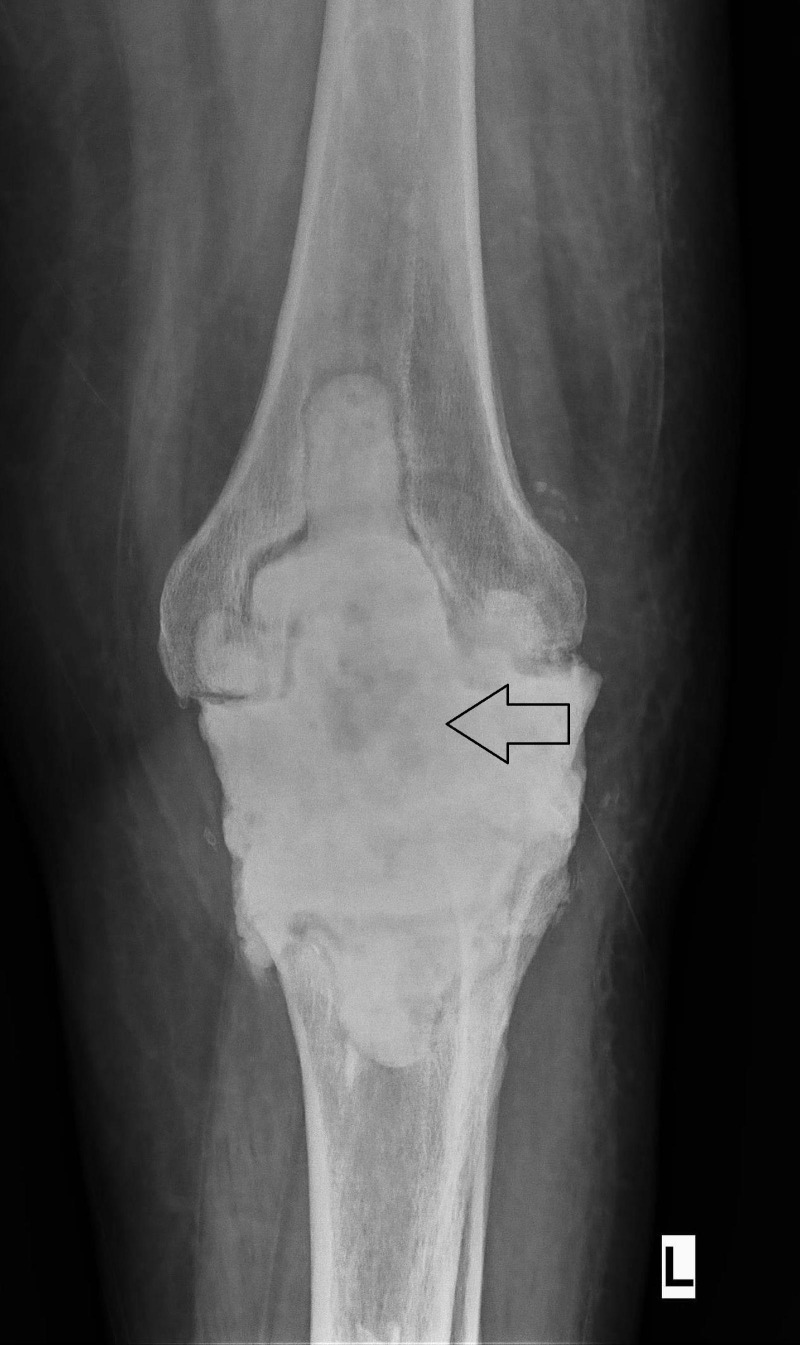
X-ray of the Left Knee Showing the Antibiotic-impregnated Bone Cement as Spacer after the Removal of Infected Implants
